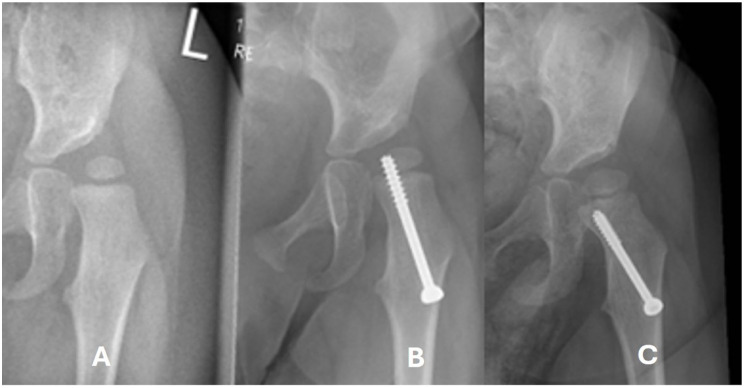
Guided growth around the hip. Radiographs are preoperative (a), direct postoperative (b), and after 14 month follow-up (c). A decrease in coxa valga and hip migration is seen. In (c), continued physeal growth means the screw threads no longer cross the physis, and screw revision is planned.
